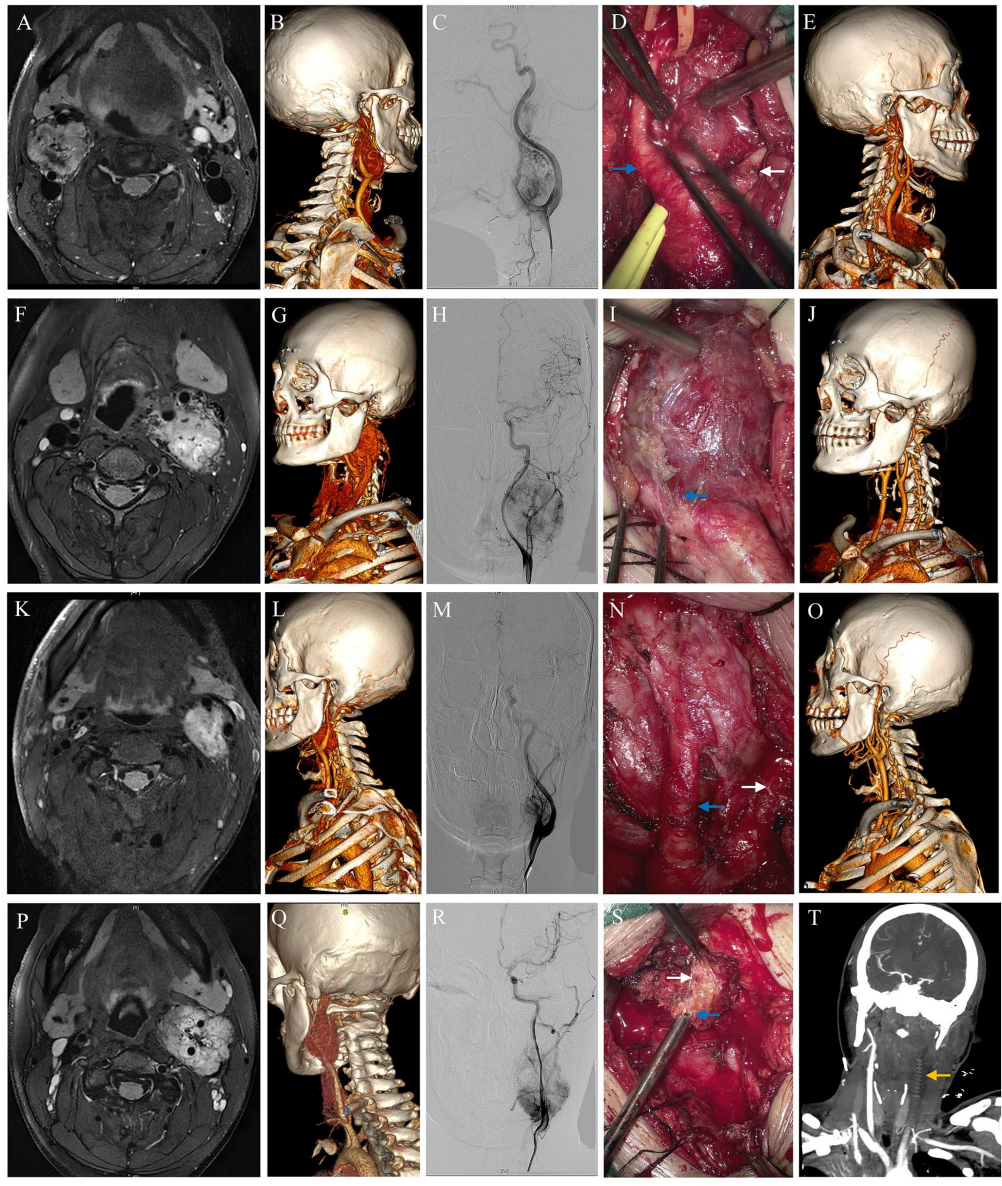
The preoperative MRI, CTA and DSA, intraoperative image and postoperative CTA images of different subtypes of tumors based on arterial relevant classification. A-E. Classical type. F-J. Medial type. K-O. Lateral type. P-T. Enveloped type. White arrow: ECA. Blue arrow: ICA. Yellow arrow: artificial vessel. The artificial vessel was used for ICA reconstruction but failed due to intravascular thrombosis
